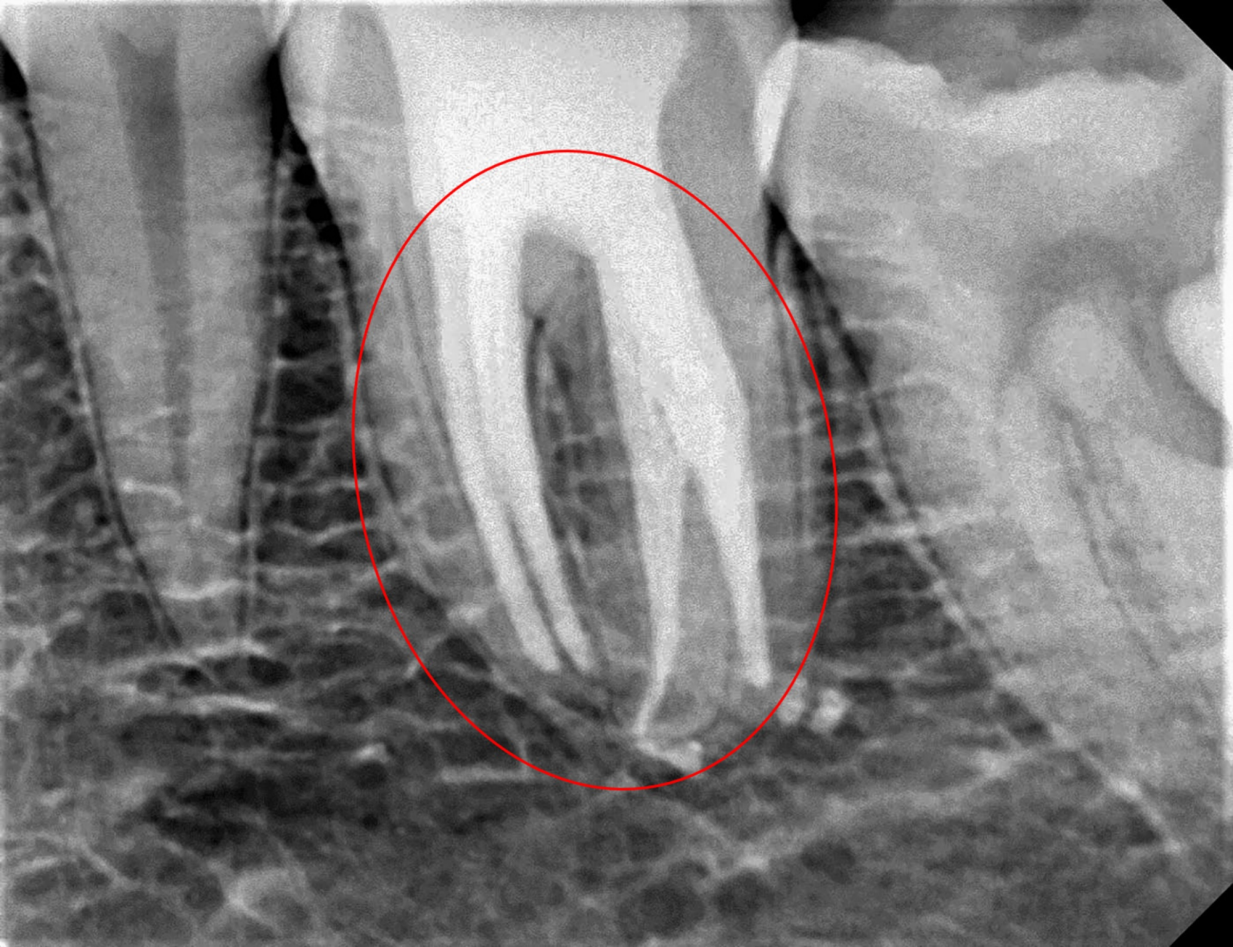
Post-obturation radiograph
